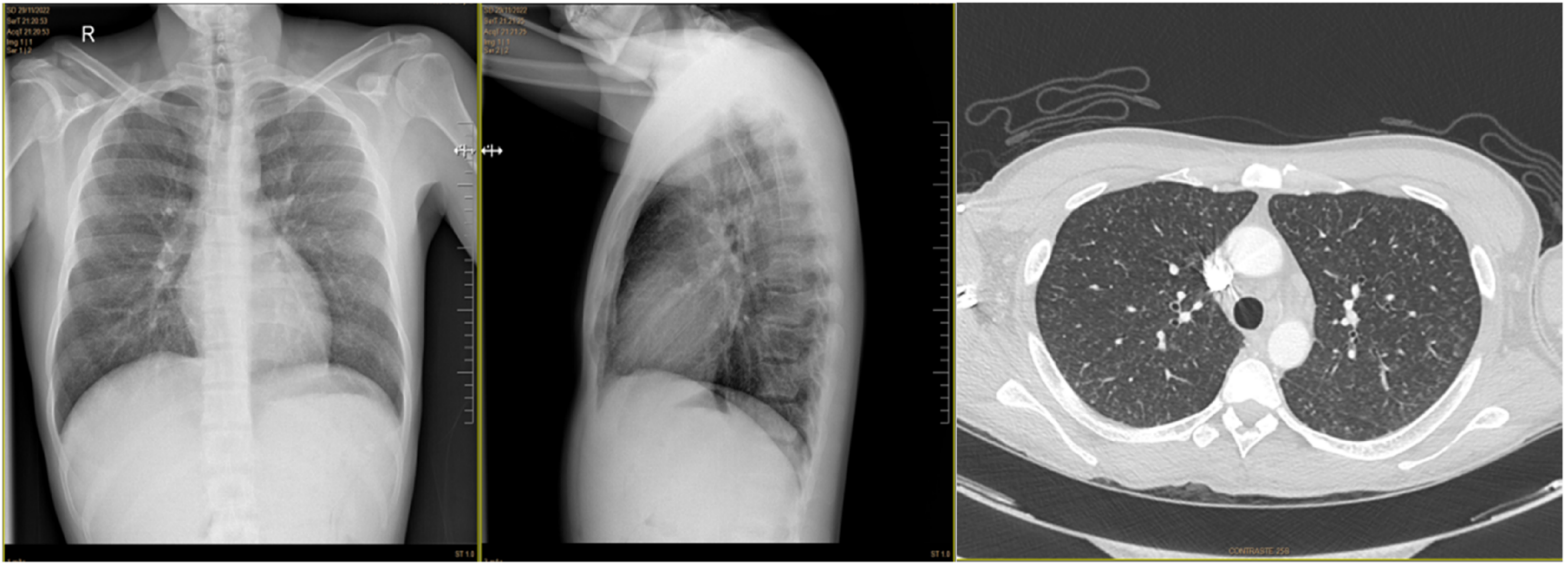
Chest computed tomography with lesions suggestive of infection.
